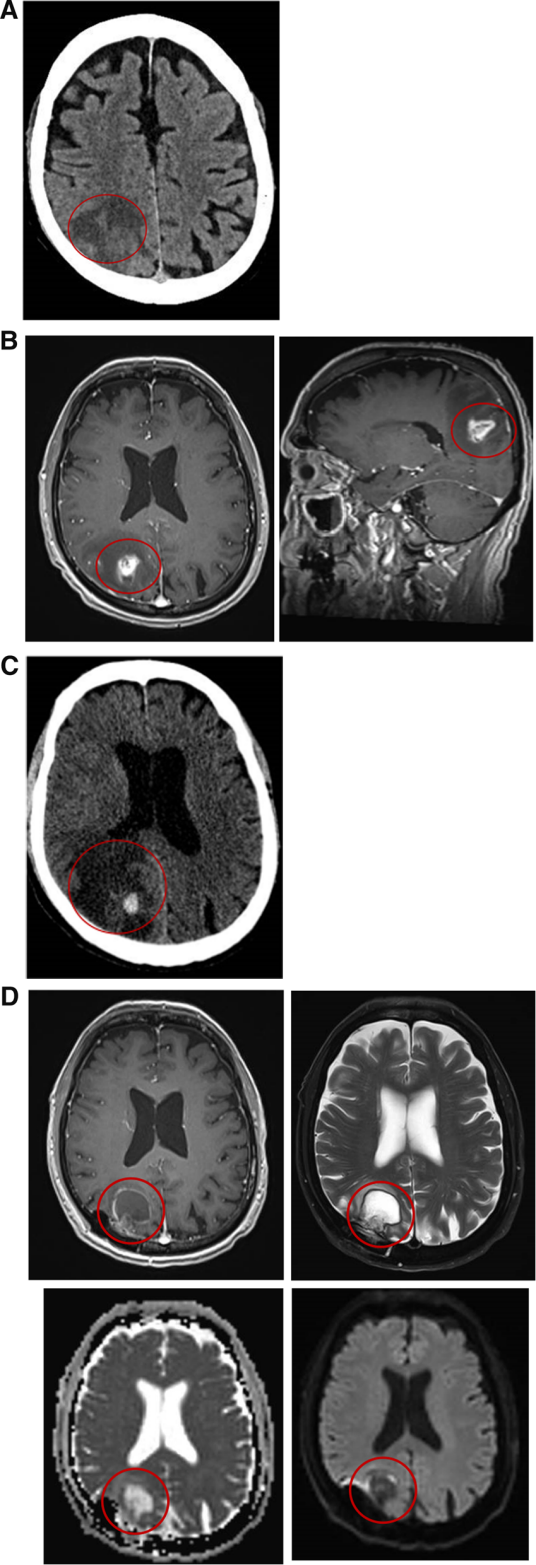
A Non-contrast CT scan showing the progress of the hypodense area in Fig. 1. B Magnetic resonance image (MRI) showing a right parietal space-occupying lesion (SOL), enhanced, surrounded by edema, with restricted diffusion in the periphery and no suspicion of bleeding on susceptibility weighted imaging. C A CT scan showing new hemorrhage in the SOL area. D  MRI axial view: Upper – post-contrast gadolinium T1WI (left) and T2WI (right) showing postoperative changes and significantly decreased edema and mass effect around the abscess. Lower – diffusion-weighted imaging/apparent diffusion coefficient showing thinner restricted diffusion on the periphery
